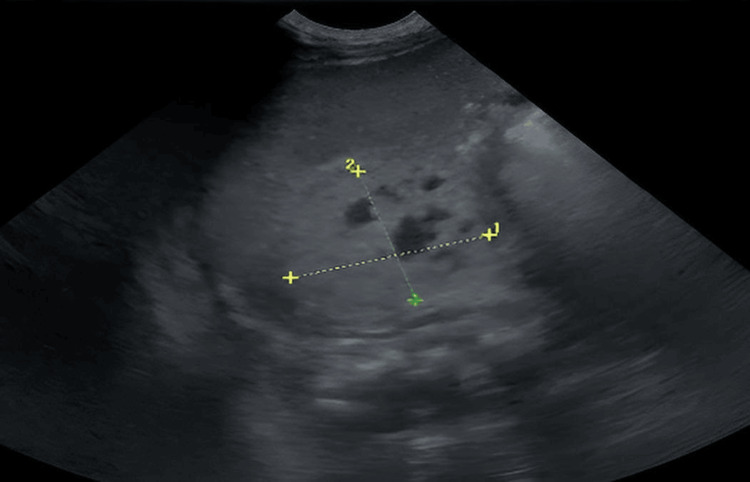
Ultrasound image of a hepatic hematoma in our patient
